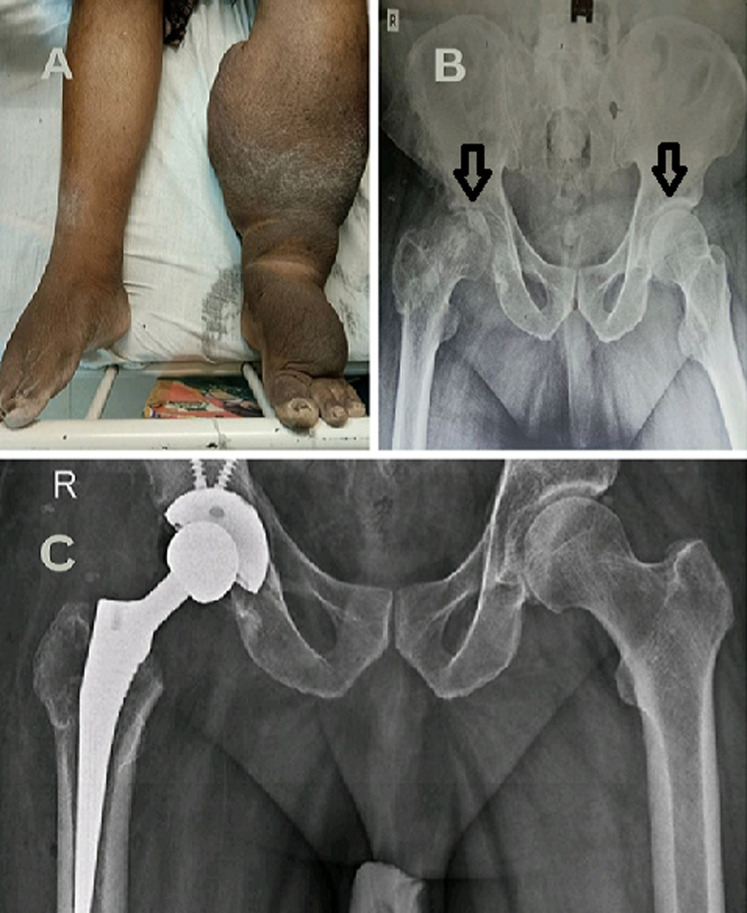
A) chronic lymphatic filariasis of bilateral lower limb (L>R); B) pre-operative Pelvic radiograph showing bilateral femoral head osteonecrosis (R>L); C) post-operative radiograph showing right total hip arthroplasty in situ
